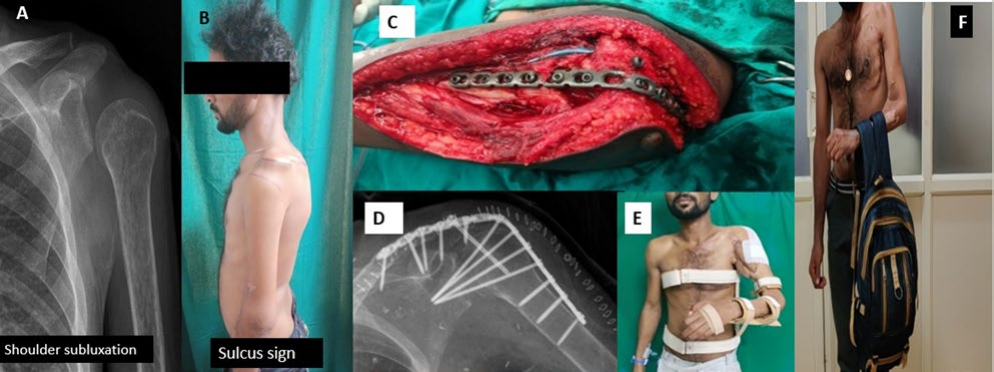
A patient with global brachial plexus palsy and an unstable shoulder. A Preoperative radiograph showing inferior subluxation of the humeral head. B Clinical photograph demonstrating a positive sulcus sign. C Intraoperative exposure of the shoulder with implant placement. D Postoperative radiograph showing screw configuration following arthrodesis. E Splint immobilization in the desired functional position. F One-year follow-up demonstrating regained shoulder stability
